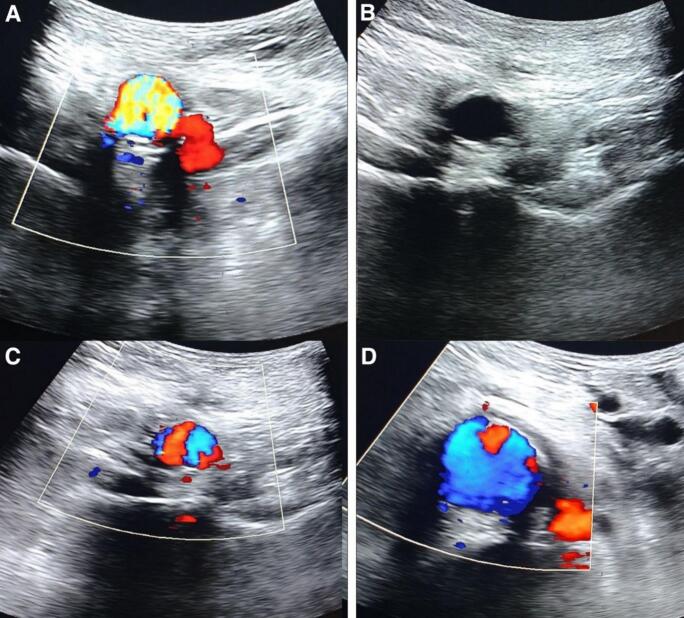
Follow-up Doppler ultrasound after 18 months demonstrates the patent graft with no complications (A&B&C&D).
